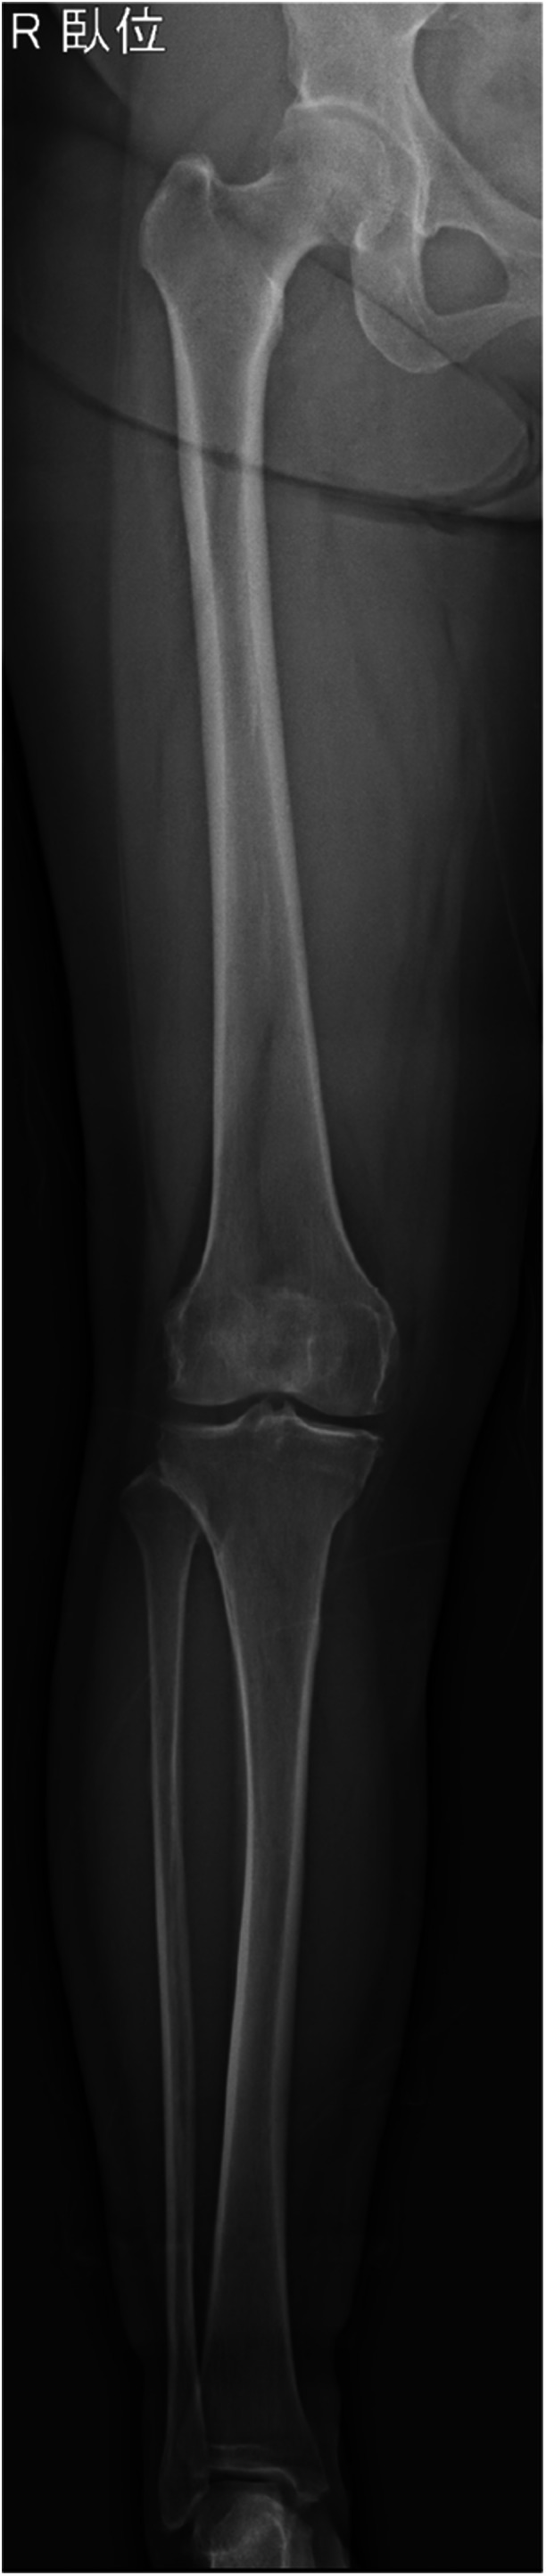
Representative full‐length lower extremity radiograph. A full‐length radiograph was taken under fluoroscopy (SONIALVISION G4, SHIMADZU, Tokyo, Japan) in the supine position, one leg at a time, with the knee fully extended and the patella facing forward. The slot radiography technique was utilized to minimize X‐ray distortion and oblique incidence, allowing for an accurate depiction of the entire lower extremity from hip to ankle in a single image.
